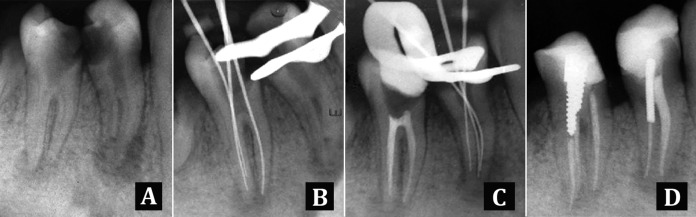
Periapical radiograph of lower premolars: A) Extensive caries and apical lesion; B) and C) working length; D) Final obturation
